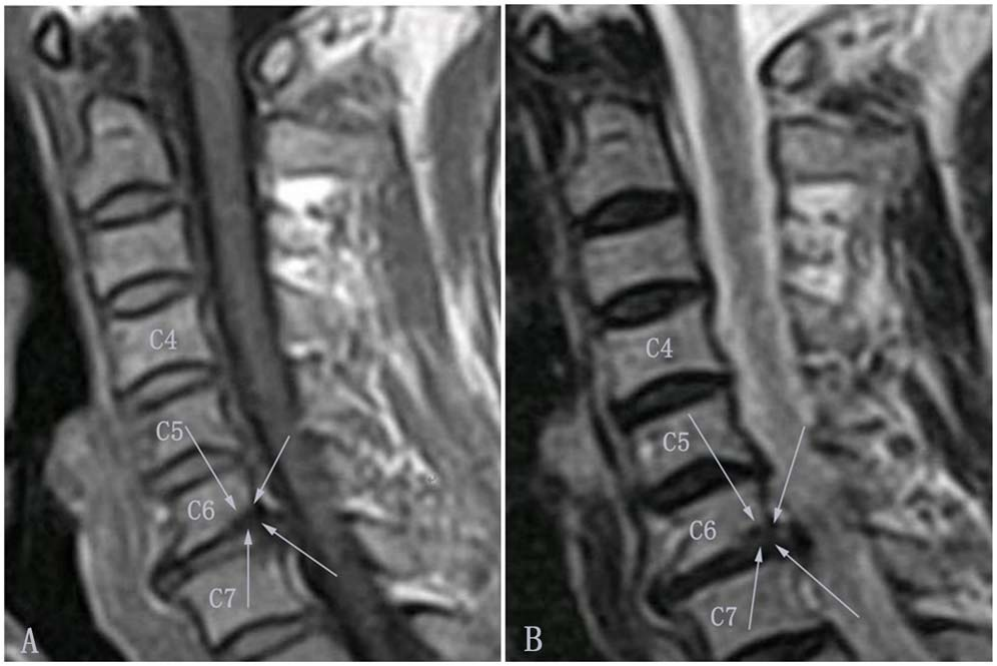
Modic type III changes in the cervical spine (indicated by arrows). Modic type III changes appear hypointense on T1-weighted (A) and hypointense on T2-weighted images (B).
